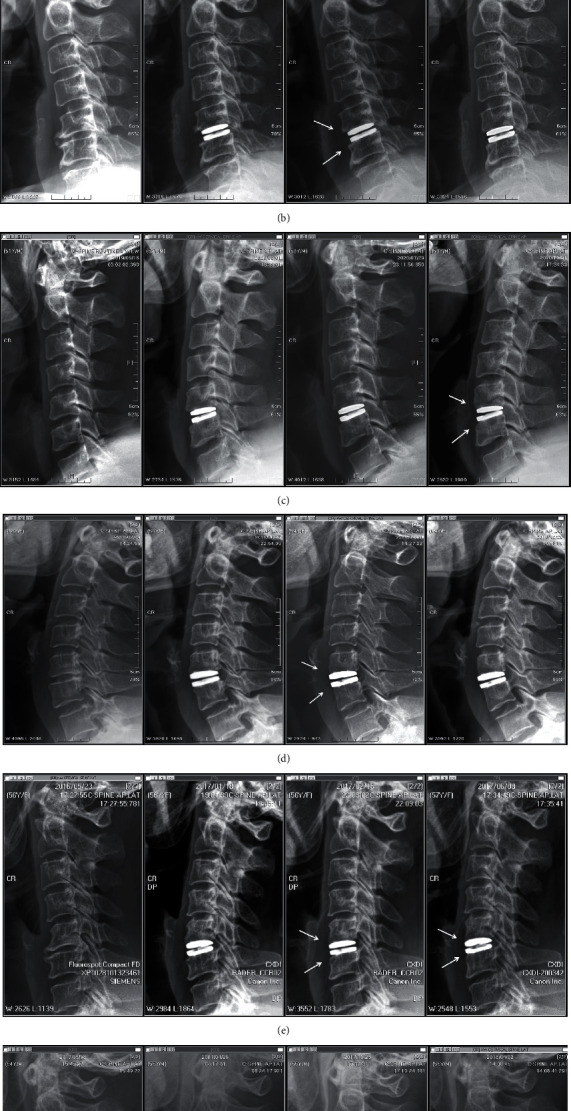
Radiographic images of different grades of anterior bone loss (ABL). (a) No ABL was noted through the follow-up period (grade 0). (b) Resorption of marginal spurs was found in both the upper and lower adjacent vertebrae at 3 months postoperation. Note that there was no change in body contour, and this condition was classified as grade 1a ABL. (c) Change in body contour was found in both the upper and lower adjacent vertebrae at 6 months postoperation. These changes were classified as grade 1b ABL. (d) Grade 2 ABL was found in the upper and lower adjacent vertebrae at 3 months postoperation. (e) Resorption of anterior marginal spurs over the upper and lower adjacent vertebrae at 1.5 months postoperation was considered grade 1a ABL. The ABL progressed by 6 months postoperation to a change in body contour (grade 1b). (f) Grade 1 ABL was noted at 6 months postoperation over C5. At 12 months postoperation, the ABL at C5 progressed to grade 2. Grade 1 ABL over C6 was also noted at 12 months postoperation.
